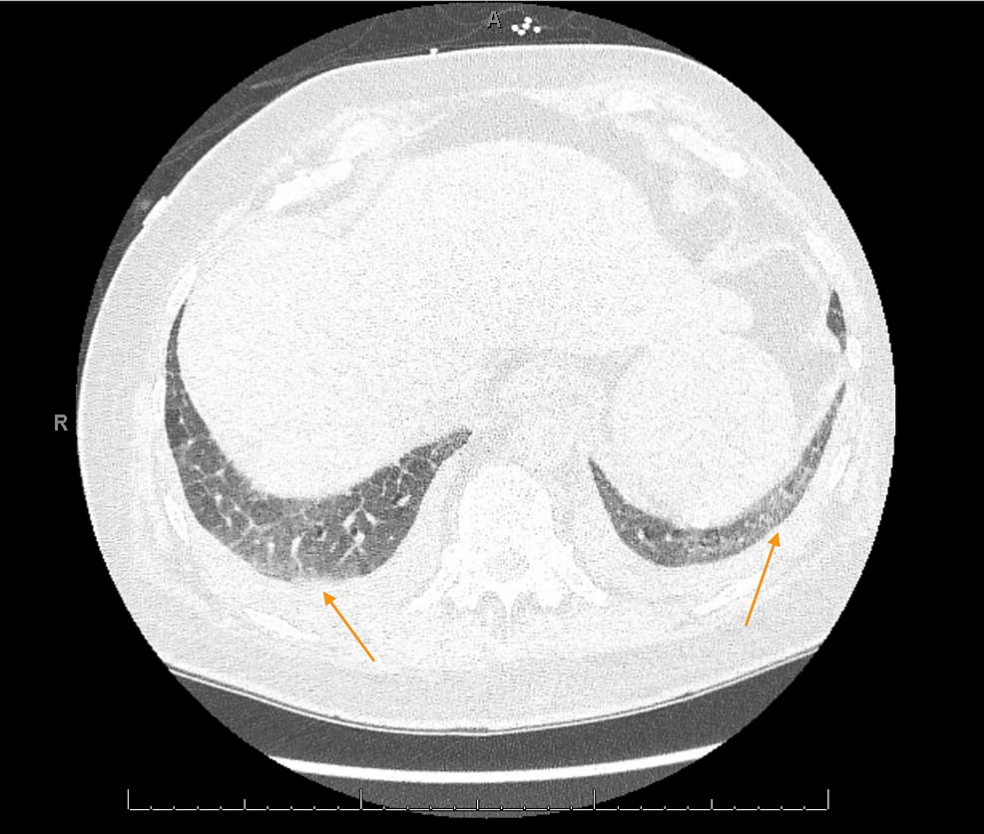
High Resolution CT Chest with Mild Bilateral Ground Glass Opacities, Lower Lobes
